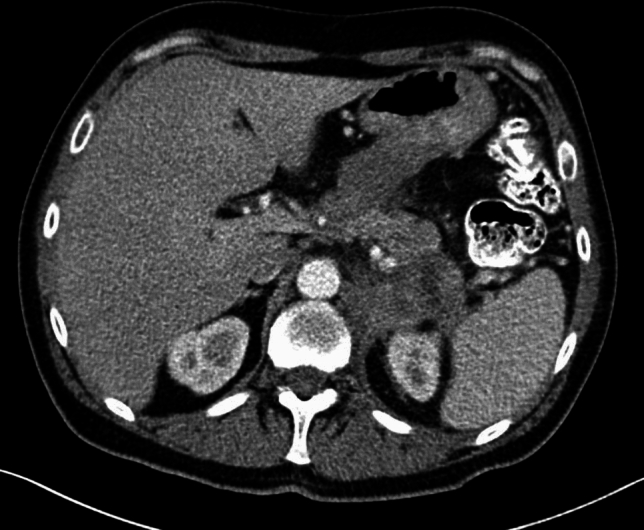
CT showing pancreatic mass taken at time of diagnosis of pancreatic cancer
